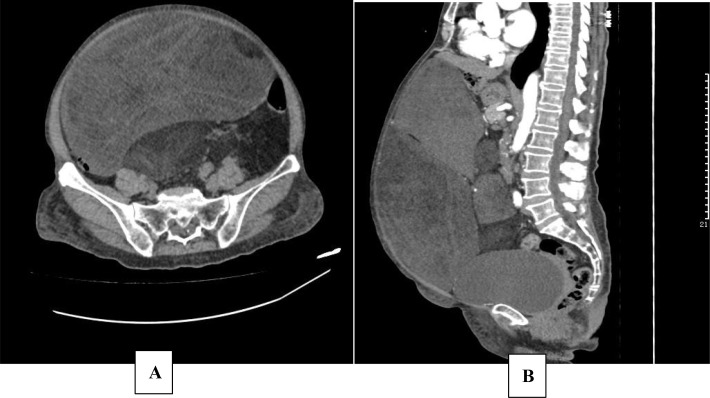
Abdominal CT. Abdominal CT obtained in April 2020 (before therapy). Axial (A); Sagittal (B).
